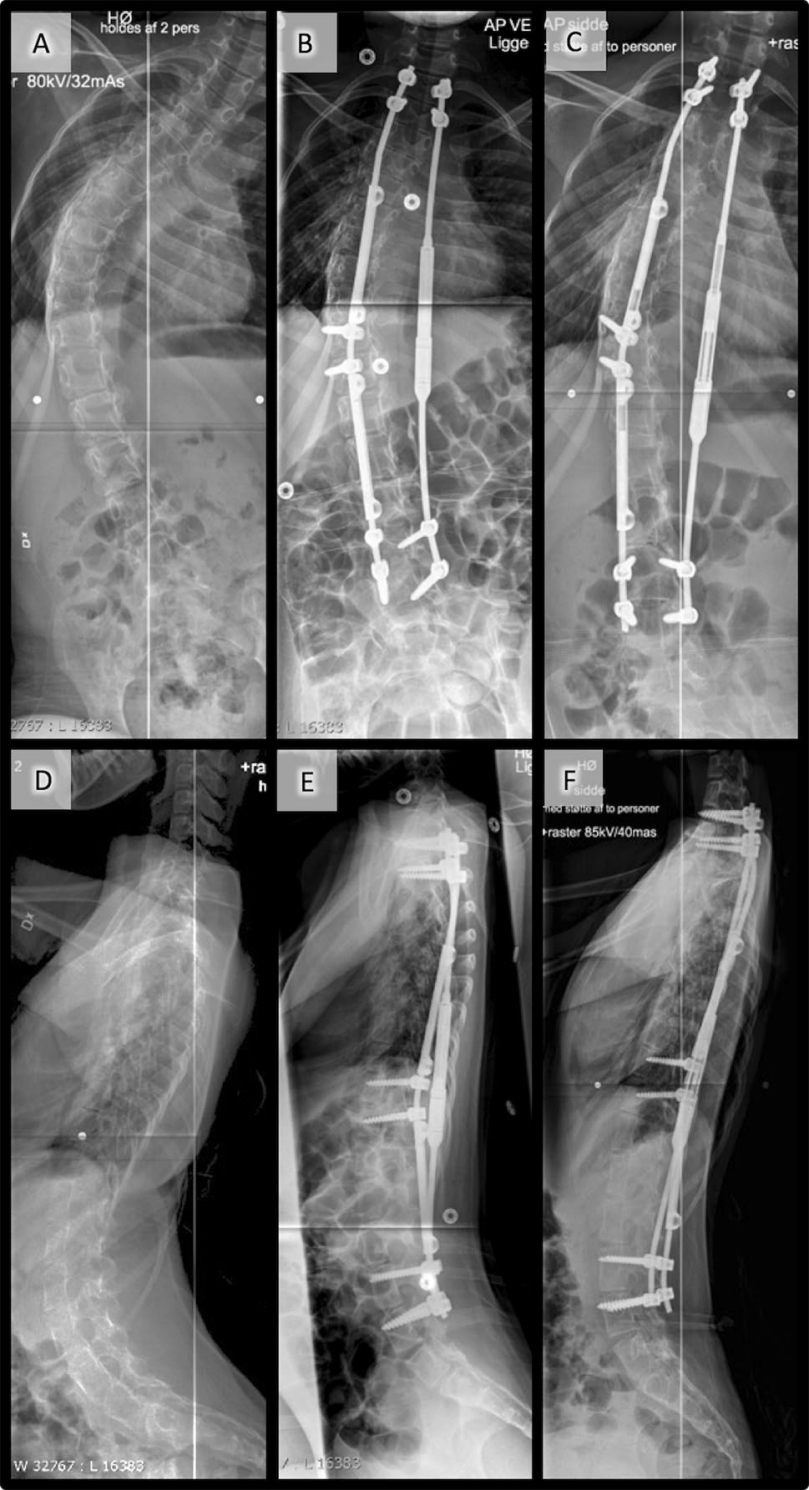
Combined single magnetic rod and CB system in an 11-year-old girl with cerebral palsy: a frontal radiographs made preoperatively, b postoperative, and c at the time of final follow-up, and d sagittal radiographs made preoperatively, e postoperative, and f at the time of last follow-up
